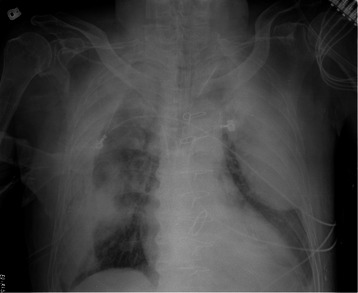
Post-operative chest plain film showing the progression of the right extrapleural hematoma. A newly developed left extrapleural hematoma was noted
